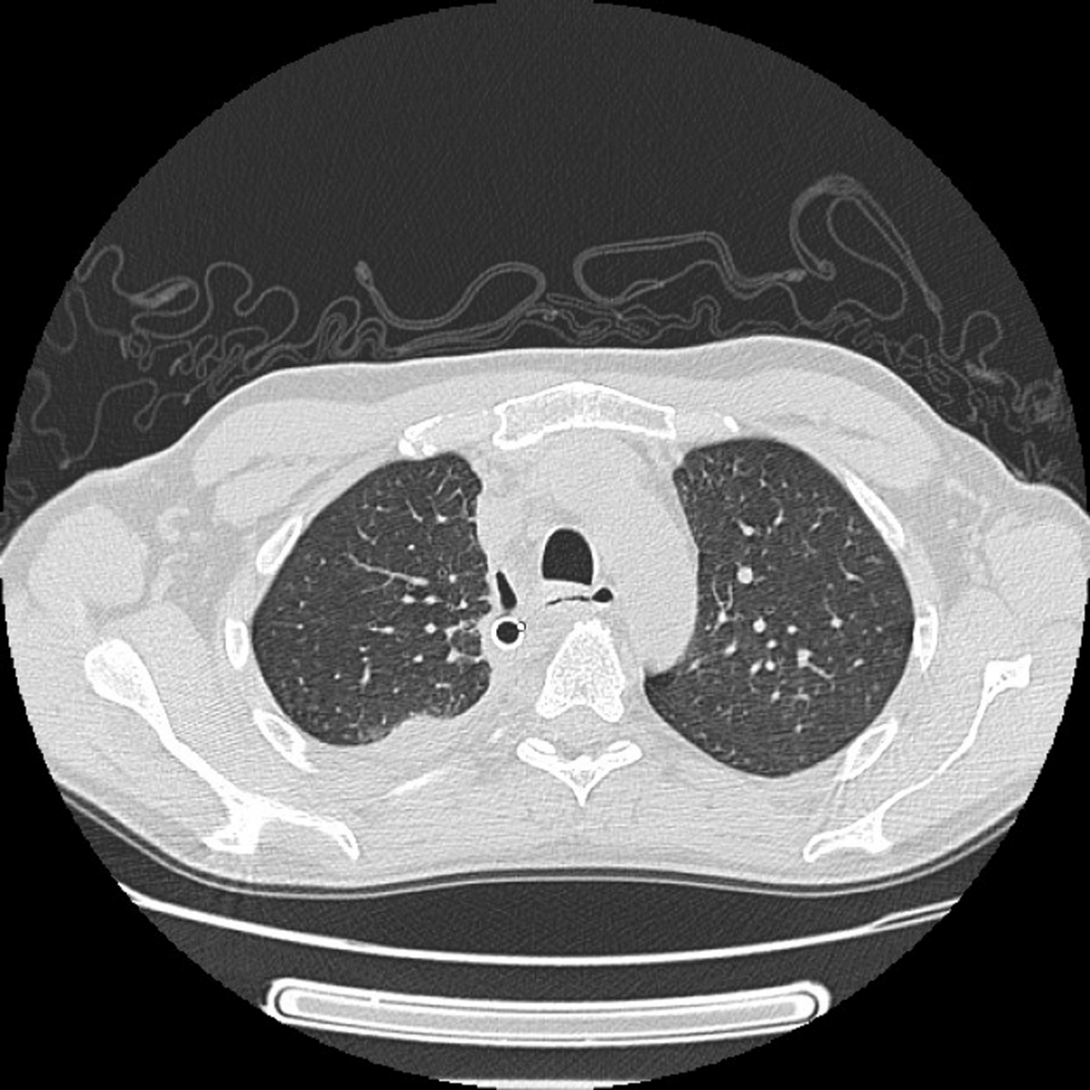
CT scan demonstrated the resolution of infections. It is well evident the chest drain within mediastinum on the right side that allowed the drainage of infection.
